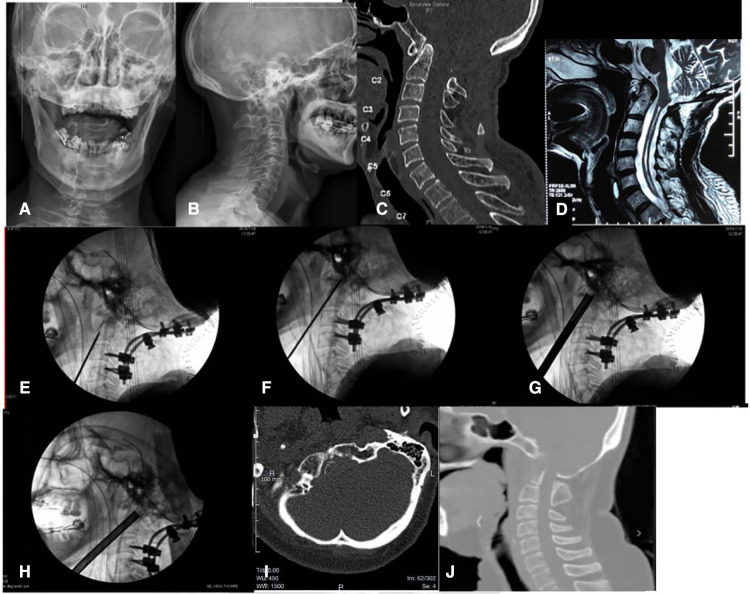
(A) AP x-ray of cervical spine of Case 2 before operation. (B) Lateral x-ray of cervical spine of Case 2 before operation. (C) Sagittal section of CT scan of Case 2 before operation. (D) Sagittal section of MR scan of Case 2 before operation. (E) The C-arm image when a Kirschner wire was drilled into the middle of the odontoid process of the axis in operation. (F) The C-arm image showed the depth of Kirschner wire reach the front of the odontoid process rear cortex and do not penetrate the rear cortex of the odontoid process. (G) The C-arm image showed a 7.2-mm hollow ring saw remove an annular bone block around the Kirschner wire. (H) The C-arm image showed lifting the end of the ring saw (the supine position) to make the angle between the ring saw and the axis of the spine larger, and removing more cancellous bone of the dentate bottom with the ring saw. (I) Cross section of CT scan after surgery. (J) Sagittal section of CT scan after surgery.
